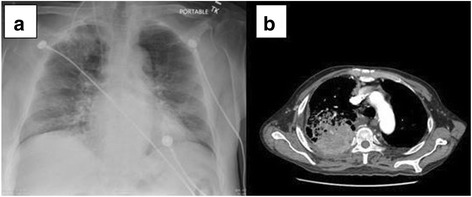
a Chest radiograph (anteroposterior view) showing the right portacath with an ill-defined right upper lobe mass. b Chest computed tomography (axial view) showing right upper lobe mass
